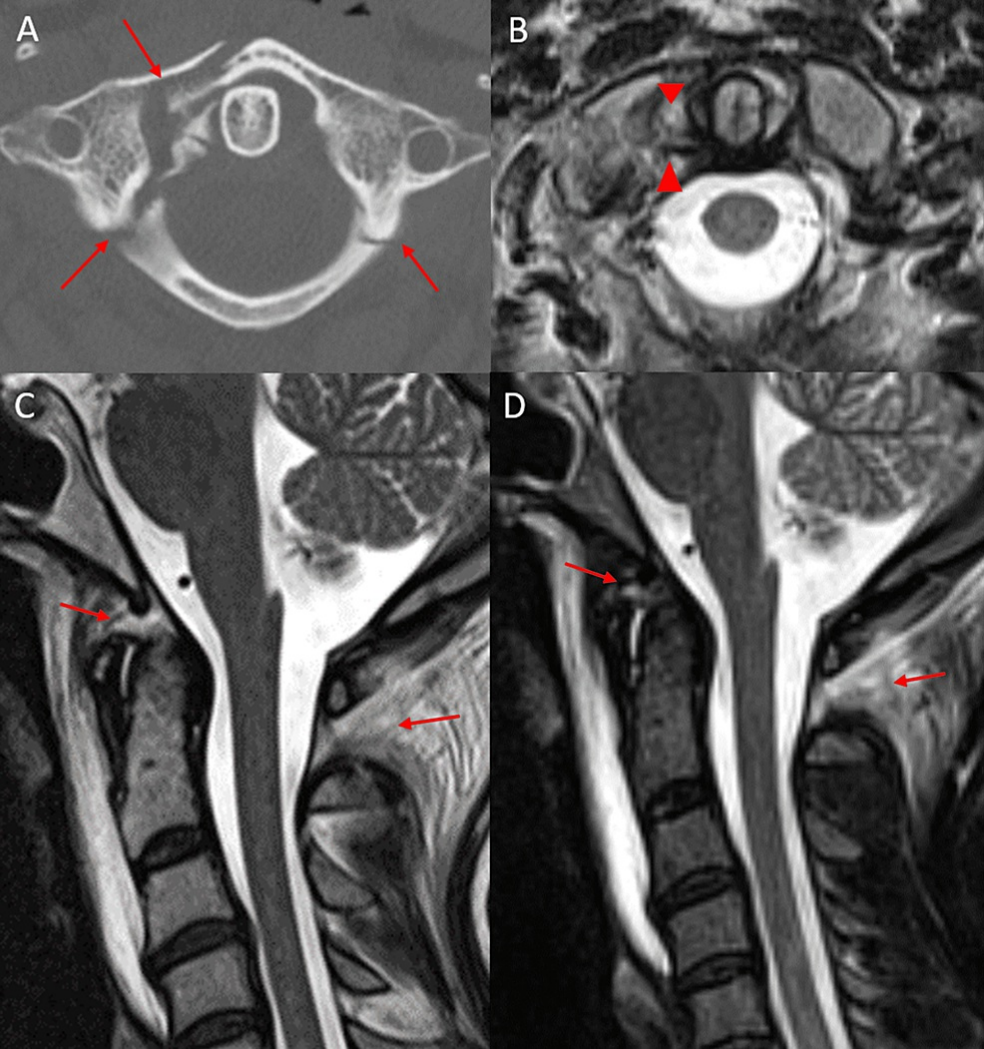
Axial CT of the cervical spine (A) in an 18-year-old trauma patient status post MVA demonstrating comminuted, right C1 anterior arch fracture and bilateral posterior arch fractures (red arrows). Axial T2-weighted MRI (B) in the same patient demonstrating a right transverse atlantal ligament tear (red arrowheads). Sagittal T2-weighted (C) and STIR-weighted (D) MRI of the cervical spine in the same patient demonstrating anterior and posterior atlanto-occipital membrane complex injuries (red arrows) with otherwise intact major craniocervical junction ligaments. Anterior and posterior atlanto-occipital membrane complex tears were relatively common in the setting of transverse atlantal ligament tears. CT: computed tomography; MVA: motor vehicle accident; MRI: magnetic resonance imaging; STIR: short tau inversion recovery
